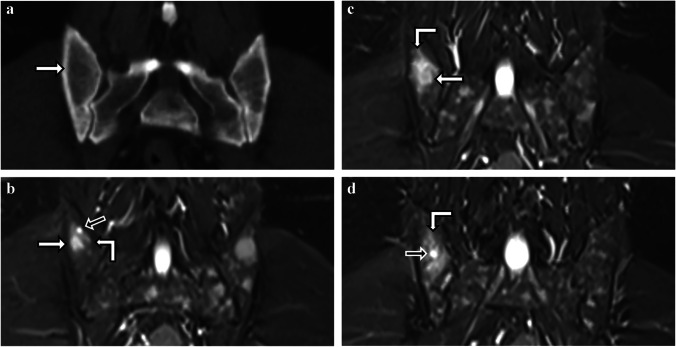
a–d Case 4. A 51-year-old male with multiple myeloma presenting with a marked discrepancy between bone marrow trephine and bone marrow aspirate results. a Coronal CT image of a scan performed one month prior to the wb-MRI scan showing a 1.0 cm by 0.7 cm osteolytic lesion in the right posterior iliac bone, which is the typical location for an unguided bone marrow biopsy (white arrows). b-d Three consecutive coronal STIR MR images from posterior to anterior of the wb-MRI show a small circular area with increased signal in STIR corresponding to the biopsy channel (b, d, black arrows) and a 1.1 cm by 0.9 cm focal lesion with a STIR-hyperintense signal in the corresponding location to the osteolytic lesion (b, c, white arrow). Bone marrow edema with intermediate STIR-hyperintensity surrounding the biopsy channel can be detected (right angle arrow)
